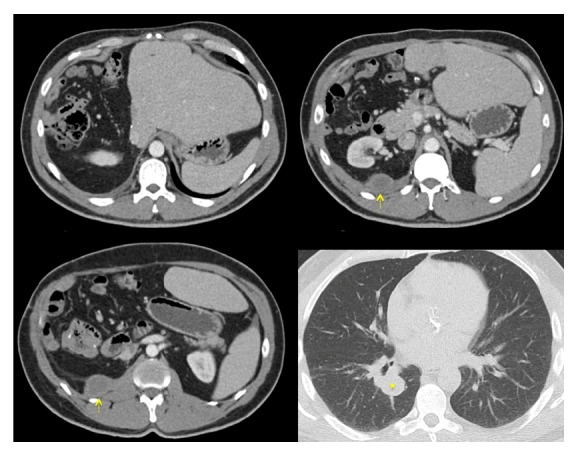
Abdomen and pelvis CT that shows a new retrocrural mass (arrows) and lower right pulmonary nodule (star). A previous right hepatectomy can be observed.
